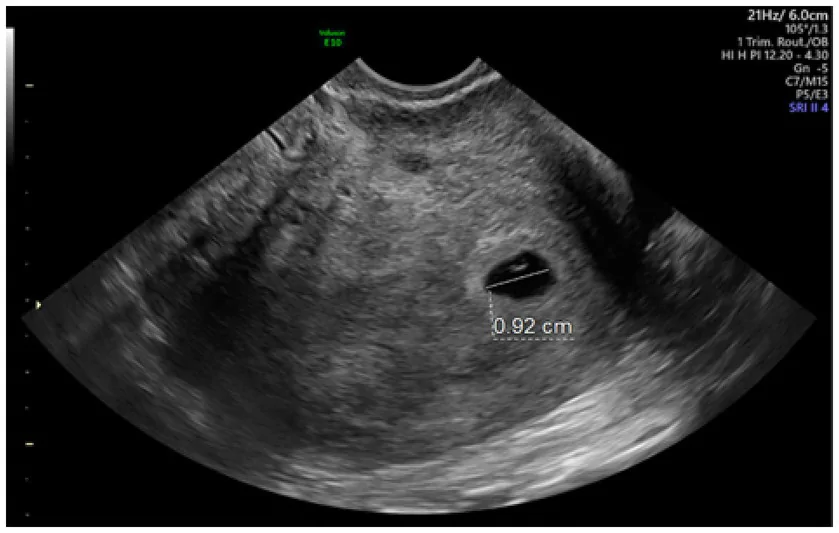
Transvaginal ultrasonographic image showing an intrauterine gestational sac with a gestational sac diameter of 9.2 mm and a yolk sac within the gestational sac, confirming an early intrauterine pregnancy. The gestational sac diameter corresponded to a gestational age of 5^+5^ weeks.
